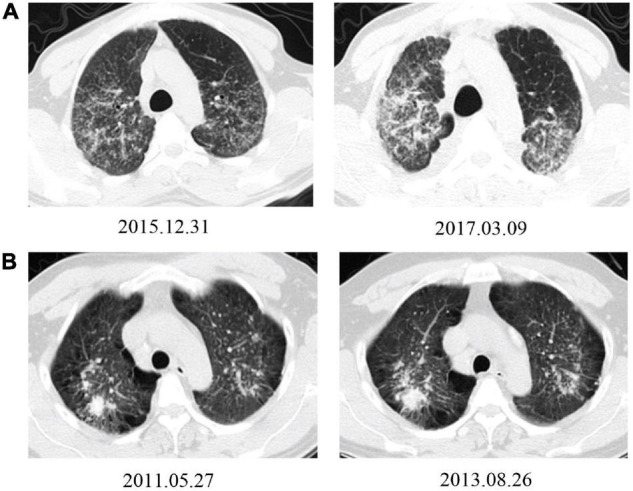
Typical HRCT images show progression from patients working with AS and non-AS. (A) A 36-year-old man had been working in AS cutting and home installing for 6 years. The HRCT image from the first registration (upper-left) and rapid progression after 15 months follow-up, with an increase of small nodules in both lung lobes; some of them connected into pieces, with pleural adhesions (upper-right). (B) A 40-year-old man had been working in metal mining for 7 years. The HRCT images from the first registration (lower-left) and slow radiological progression after 27 months follow-up period with an slightly enlargement in the upper right lung mass, increment in emphysema and bullae, enlargement and calcification in mediastinal lymph nodes (lower-right).
